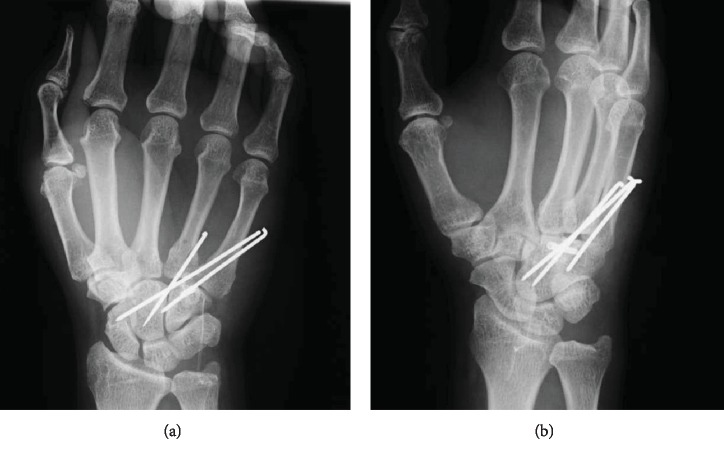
Postoperative radiograph. (a) Posteroanterior view. (b) Oblique semisupinated view.
